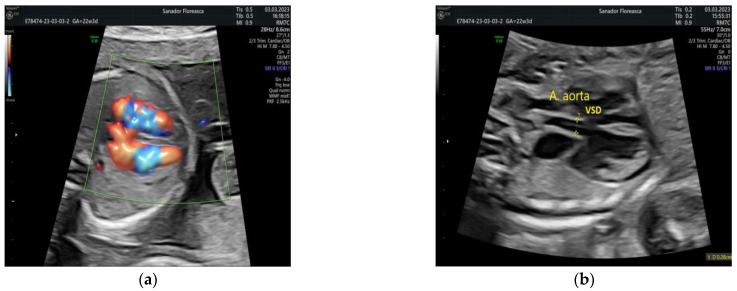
Four-chamber view in Color Doppler (a) showing a slight ventricular disproportion and the perimembranous VSD. Five-chamber view (b) showing in addition to the VSD, a small ascending aorta. VSD, ventricular septal defect.
